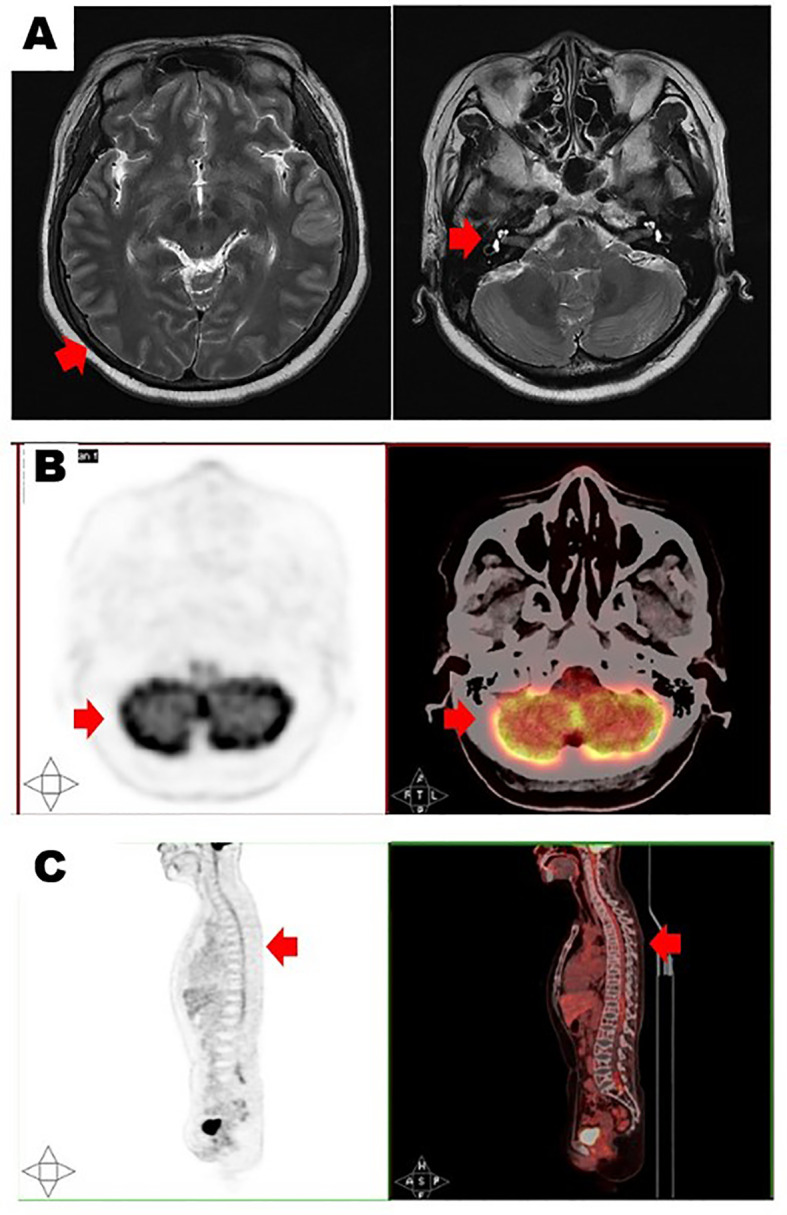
Brain magnetic resonance imaging (A) showed cerebrospinal meninges (left, red arrow) and auditory nerve thickening (right, red arrow). Positron emission tomography/computerized tomography in transverse section (B) and longitudinal section (C) showed multiple cerebrospinal meninges with increased 18F-flurodeoxyglucose metabolism (red arrows).
